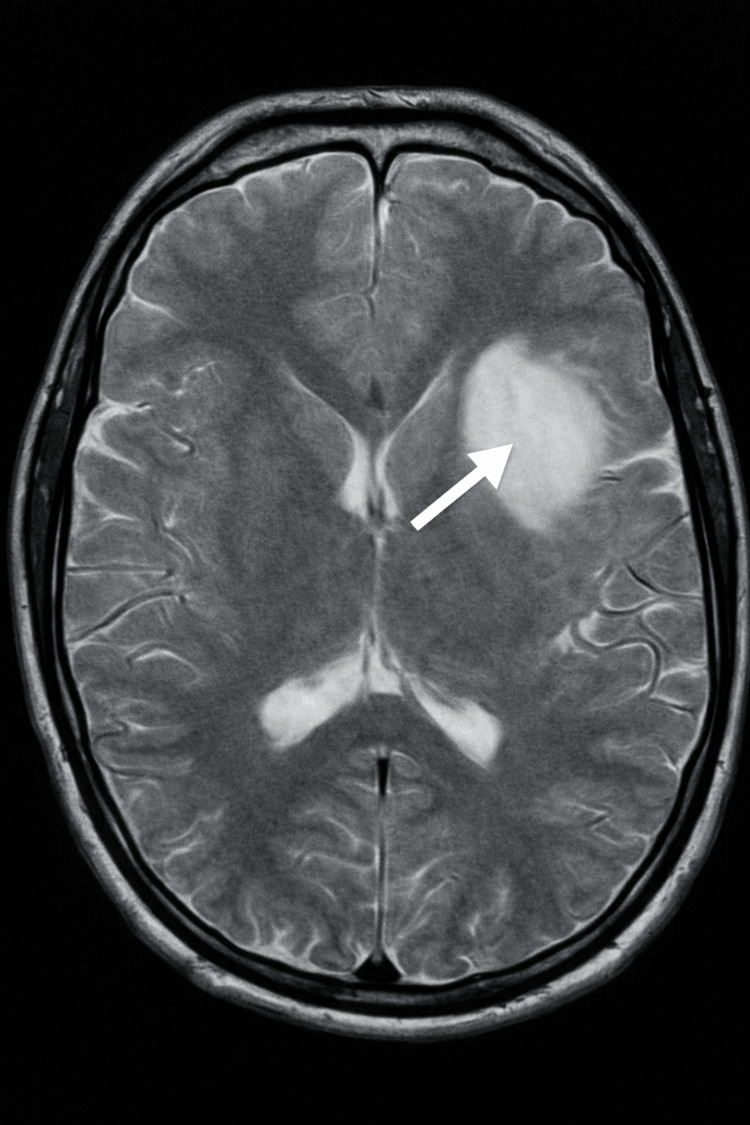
Axial T2-weighted MRI of the brain demonstrates a T2-hyperintense lesion in the left anterior external capsule extending into the anterior insular cortex and frontal white matter (arrow), with imaging features consistent with a low-grade glioma MRI: magnetic resonance imaging
